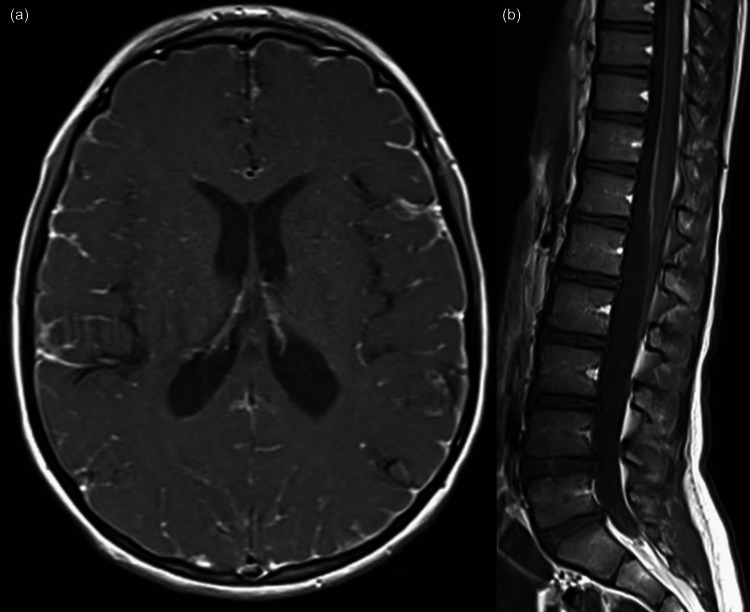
(a) MRI brain showing enhancement of leptomeninges and nonspecific signal changes in left frontal periventricular white matter. (b) MRI spine showing enhancement and focal thickening dorsal to the spinal cord at lower thoracic levels
